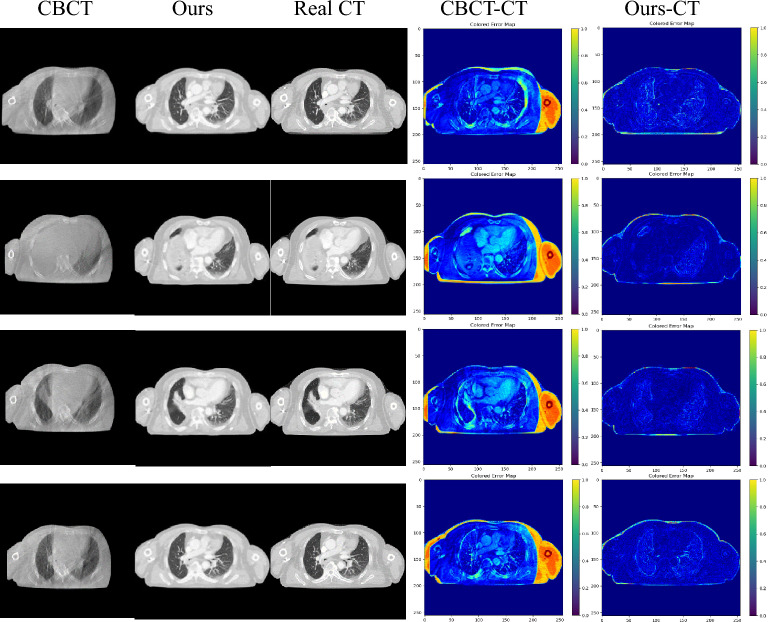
Error plots of CBCT vs. CT and the method of this paper vs. CT under the Chest dataset.
